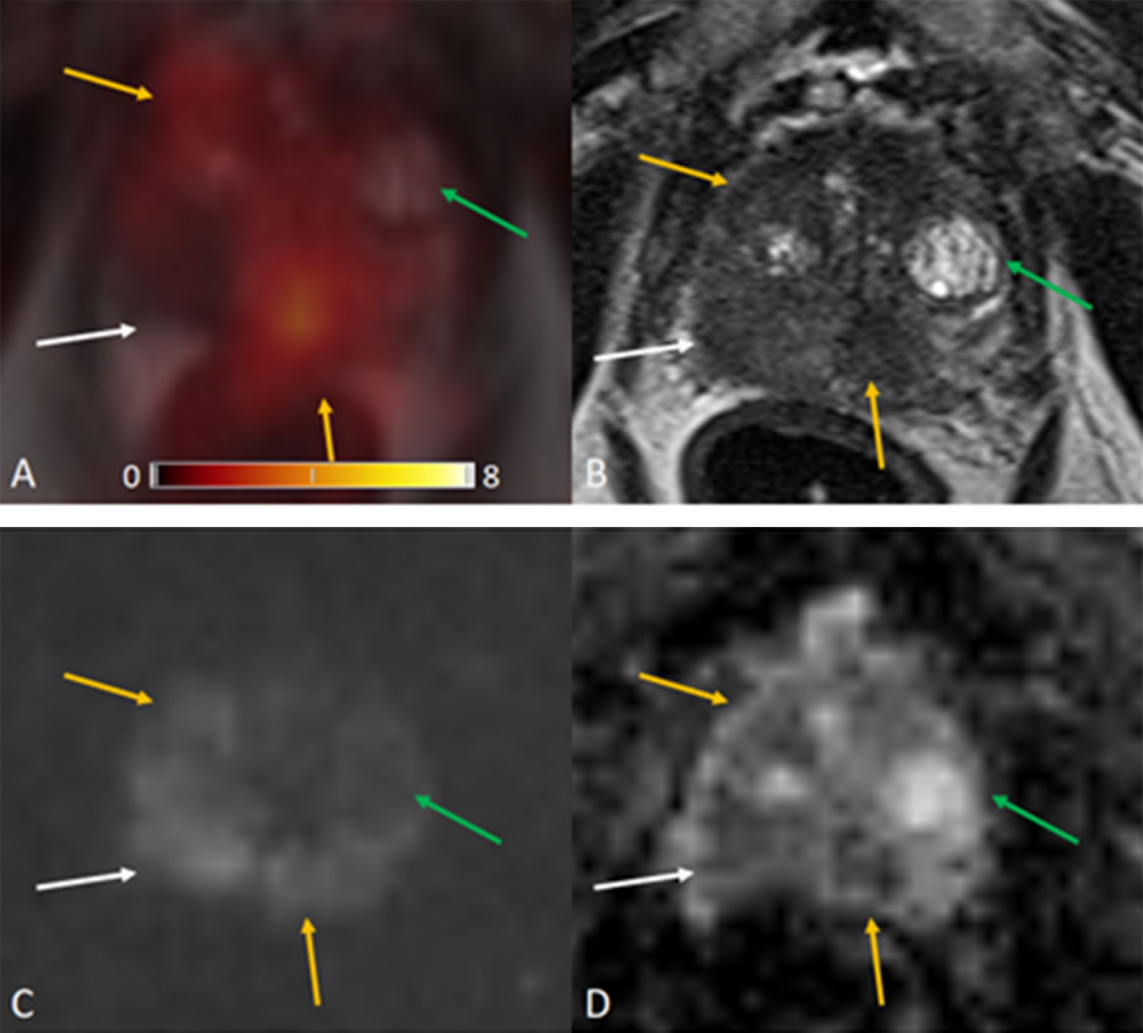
[^68^Ga]Ga-PSMA PET/MRI images obtained in a 79-year-old man, PSA 2.20 ng/mL. a [^68^Ga]Ga-PSMA PET/MRI; b T2WI; c DWI, *b* value 1000 s/mm^2^; d ADC. Background SUVmax 1.7. MRI-positive lesions are yellow arrows and white arrow. Yellow arrow: SUVmax 5.9, LBR 3.5 (middle of PZ) and 4.2, LBR 2.5 (right TZ). White arrow: SUVmax 1.6, LBR 0.9 (right PZ). MRI-negative lesion is green arrow, SUVmax 1.4, LBR 0.8 (left TZ). This example showed that MRI-positive lesions could be either PSMA avid uptake (yellow arrows) or unapparent PSMA uptake (white arrows). And MRI-negative lesions could be unapparent PSMA uptake (green arrows)
